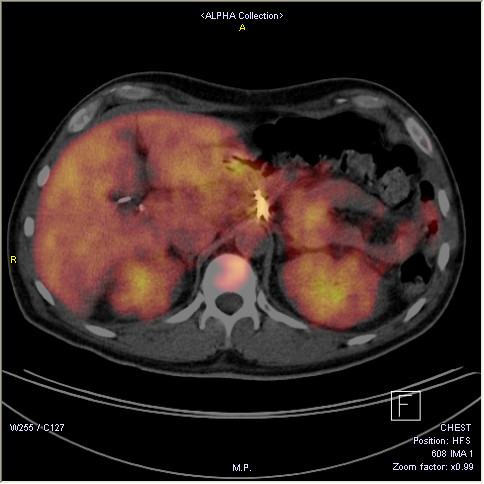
Abdominal PET/CT. Figure 2. PET/CT fusion image taken six months into treatment with erlotinib and corticosteroids showing postoperative findings only. There is no increased uptake of ^18^F-FDG.
